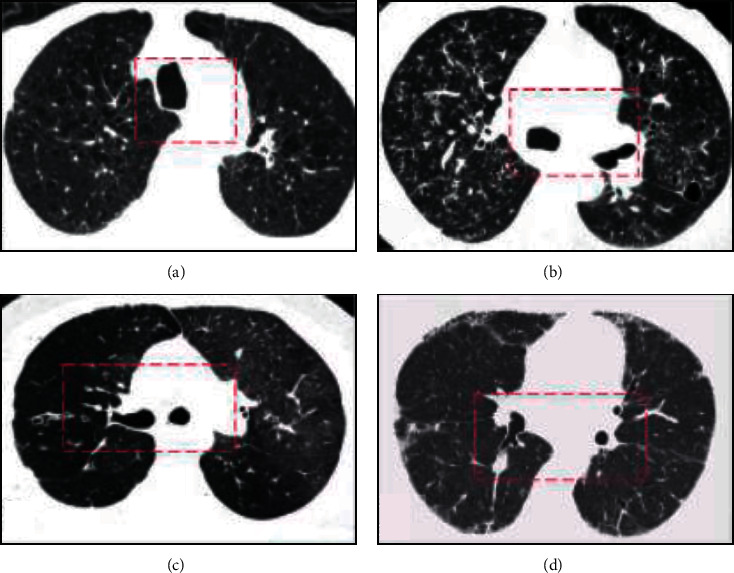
HRCT images of asthmatic children with small airway obstruction. (a) Bronchiolar wall thickening; (b) tree-in-bud; (c) air trapping; (d) mosaic sign.
